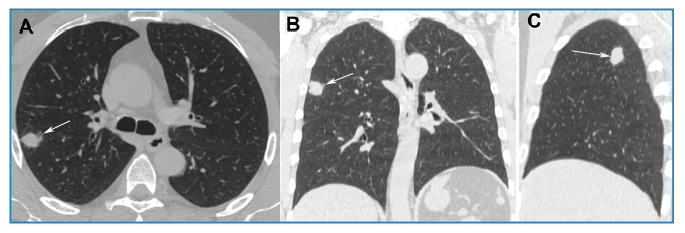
Non-enhanced chest CT images with axial (A), coronal (B), and sagittal (C) reconstruction reveal a nodule with homogenous soft-tissue density, irregular contours, and a maximum diameter of approximately 19 mm in the upper lobe of the right lung (arrows).
